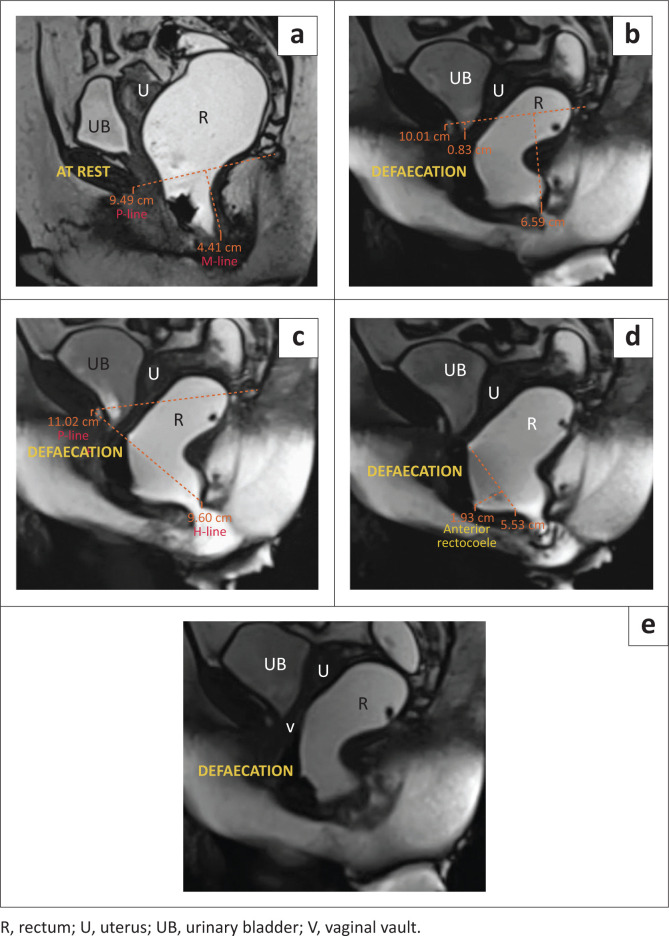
(a–e) MRI defaecogram: Grade 3 anorectal descent with anterior rectocoele. (a) At rest shows Grade 2 anorectal descent. (b) The defaecation phase shows Grade 3 anorectal descent with a small cystocoele. (c) During defaecation an increased H-line, representing levator hiatal widening is observed. (d) During defaecation a small anterior rectocoele is depicted. (e) The defaecation phase shows significant post-void residual intra-rectal jelly.
